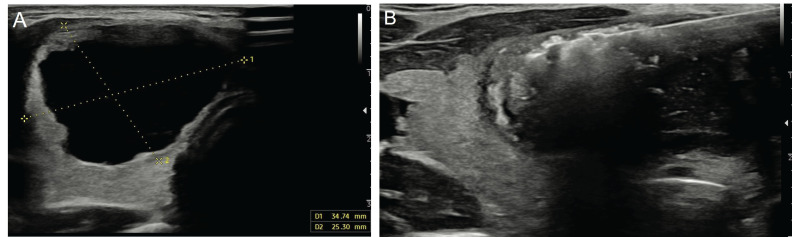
Ultrasonography image of a 28-year-old female patient shows a pure cystic nodule (A) measuring 34x25 mm in the right lobe of the thyroid gland. The patient underwent ethanol ablation (B).
